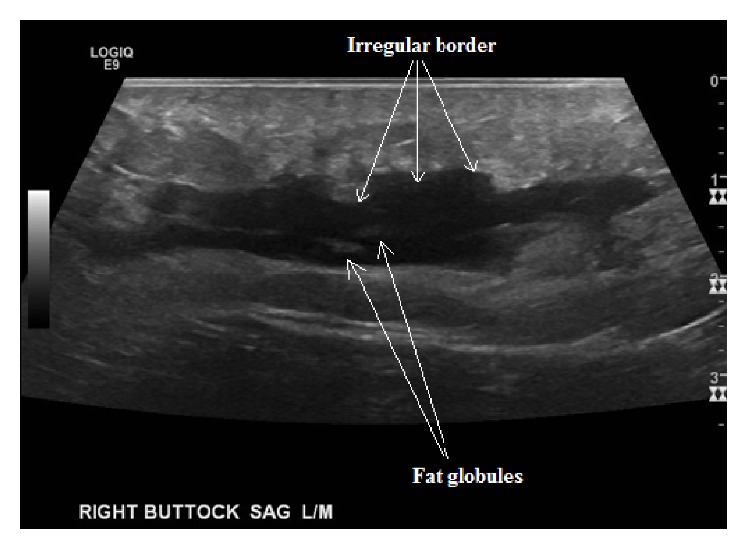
Sagittal ultrasound view of lesion. Note irregular borders and echogenic fat globules.
